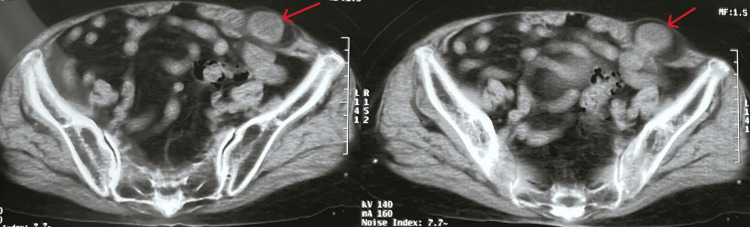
CT scan of the pelvic abdomen showing an image of Spigelian hernia in the lower left abdomen. CT, computed tomography
